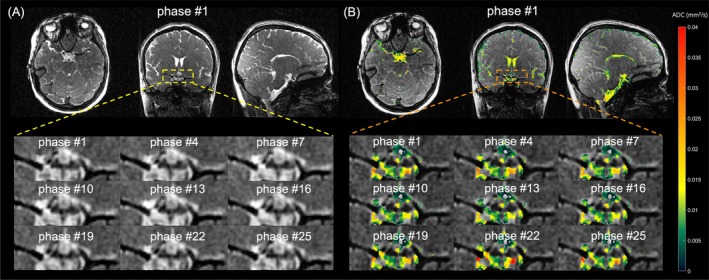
The human brain MRI results of image reconstruction and ADC quantification in one representative subject. Shown are the DECAF images (A) and quantified ADC map in the whole‐brain CSF regions overlayed on the original images (B). Images and ADC maps in every 3 phases are displayed (25 phases in total).
